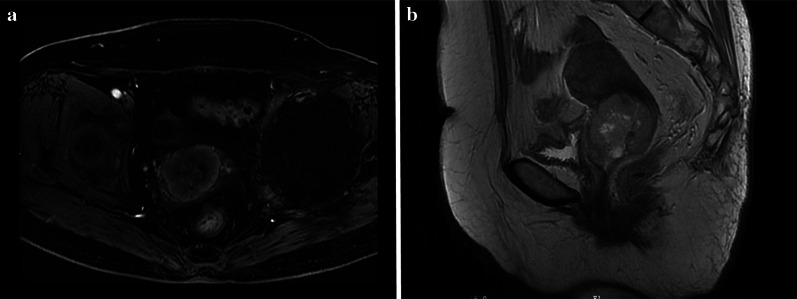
A 70-year-old woman with cervical carcinosarcoma (CCS). Sagittal T2WI magnetic resonance imaging (MRI) (a) showed a mixed signal tumor in the cavity. Axial delayed enhanced T1WI (b) showed a heterogeneous mild enhancement tumor
